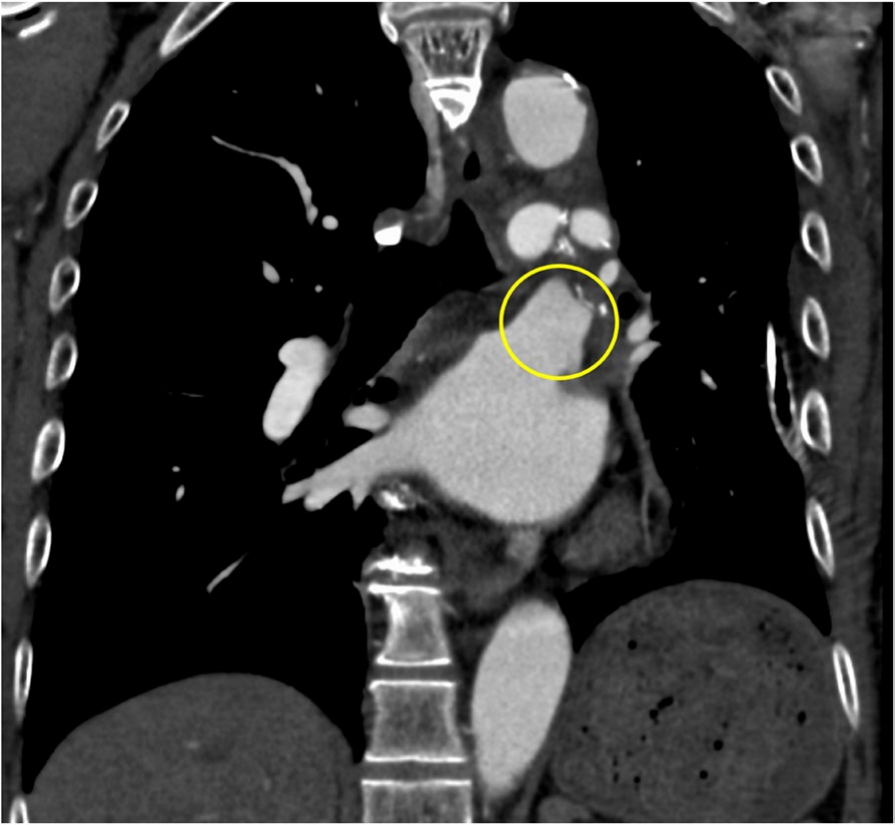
Contrast-enhanced chest computed tomography scan showing no thrombus in the stump of the left superior pulmonary vein (yellow circle)
